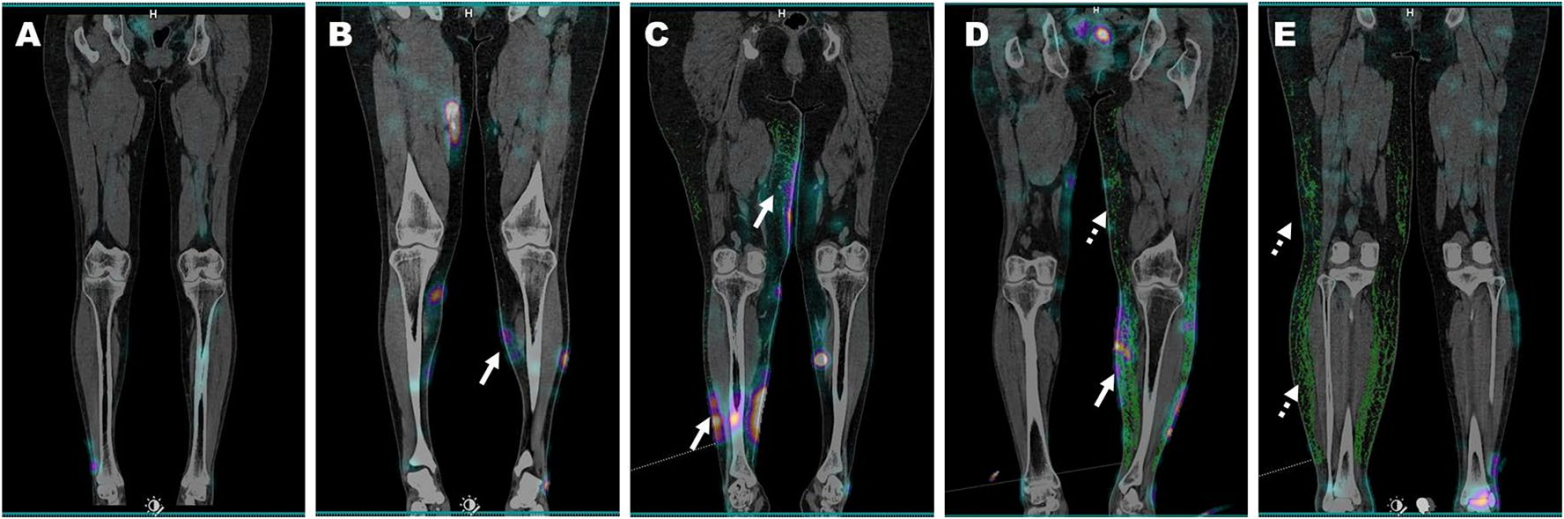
Representative cases according to the hybrid SPECT/CT classification. The coronal view of fused SPECT/CT for each case is presented, with the green area representing segmented HP from the CT image. (A) A 70-year-old male patient with lymphedema in the right lower limb. There were no observations of DBF and HP throughout the entire limb, and as a result, it was classified as Class 1 according to the hybrid SPECT/CT classification. The clinical severity was Grade 0, and the TLS stage was P-1. (B) A 54-year-old female patient with lymphedema in the left lower limb. DBF was observed in the distal limb, while HP was absent (arrow). As a result, it was classified as Class 2 according to the hybrid SPECT/CT classification. The clinical severity was Grade 0, and the TLS stage was P-2. (C) A 68-year-old female patient with lymphedema in the right lower limb. Both proximal and distal limbs showed the presence of DBF accompanied by HP (arrows), and as a result, it was classified as Class 3 according to the hybrid SPECT/CT classification. The clinical severity was Grade 1, and the TLS stage was P-3. (D) A 63-year-old female patient with lymphedema in the left lower limb. In the distal limb, DBF accompanied by HP was observed (arrow), while in the proximal limb, HP without DBF was observed (dotted arrow). As a result, it was classified as Class 4 according to the hybrid SPECT/CT classification. The clinical severity was Grade 4, and the TLS stage was T-4. (E) A 60-year-old female patient with lymphedema in the right lower limb. Throughout the entire limb, HP was observed without DBF (dotted arrows), and as a result, it was classified as Class 5 according to the hybrid SPECT/CT classification. The clinical severity was Grade 3, and the TLS stage was T-6. *DBF* dermal backflow, *HP* honeycomb pattern, *TLS* Taiwan Lymphoscintigraphy Staging.
